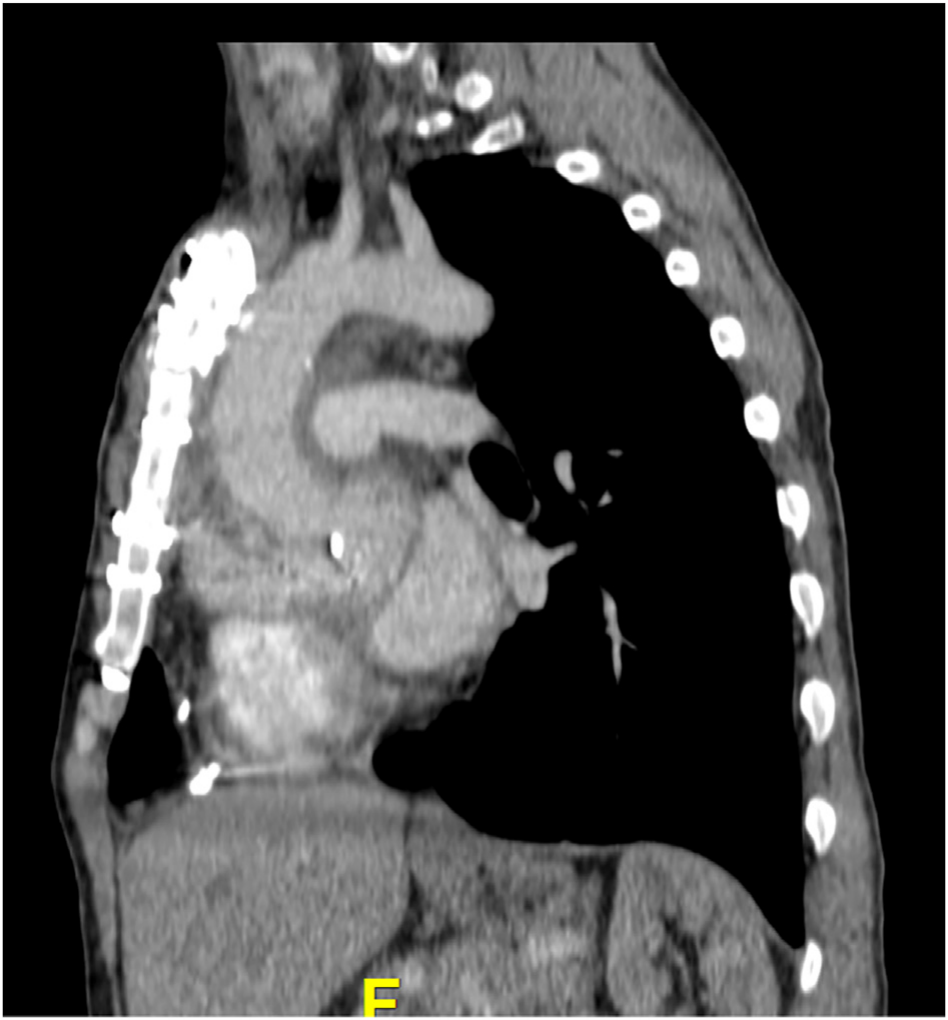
Preoperative computed tomography scan showing proximity of the ascending aorta to the sternum.
